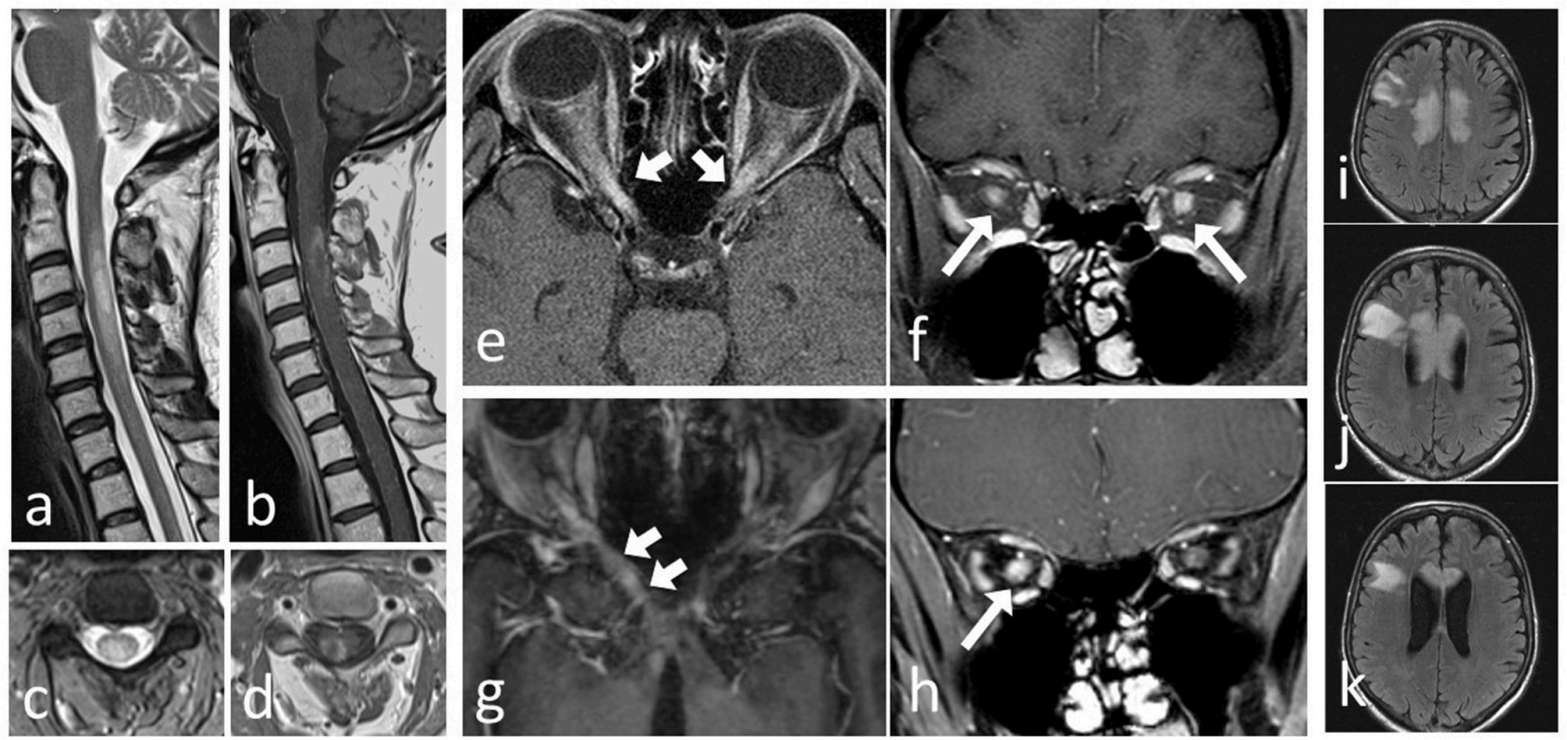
MRI of patients with AQP4-Ab positive NMOSD and patients with MOG-EM. Severe cervical LETM in a NMOSD AQP+ patient: (a) T2 sagittal and (b) T2 axial of a cervical myelon lesion with ring Gd-Enhancement and T1 hypointense center in (c) T1+Gd sagittal and (d) T1+Gd axial. Bilateral opticusneuritis in a MOG-EM patient (e) T1+Gd axial and (f) T1+Gd coronar. Unilateral optic neuritis with chiasmal involvement in a NMOSD AQP4+ patient: (g) T1+Gd axial and (h) T1+Gd coronar. Tumefactive lesion involving the corpus callosum in a NMOSD AQP4+ patient (i–k) T2 axial.
